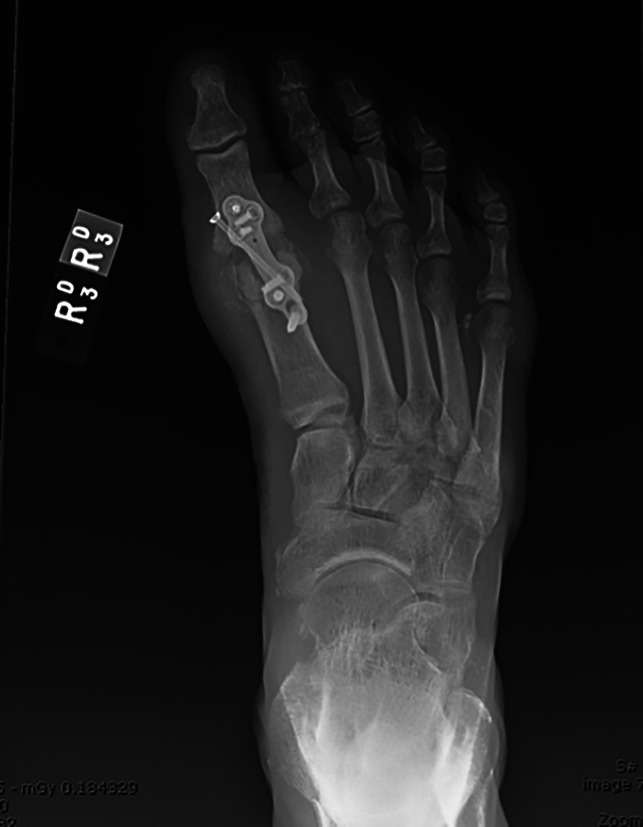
Postoperative radiographic imaging showing removal of tophi, first MTP joint deformity correction, and second PIP joint resection arthroplasty (author's clinical photograph). MTP = metatarsophalangeal, PIP = proximal interphalangeal
